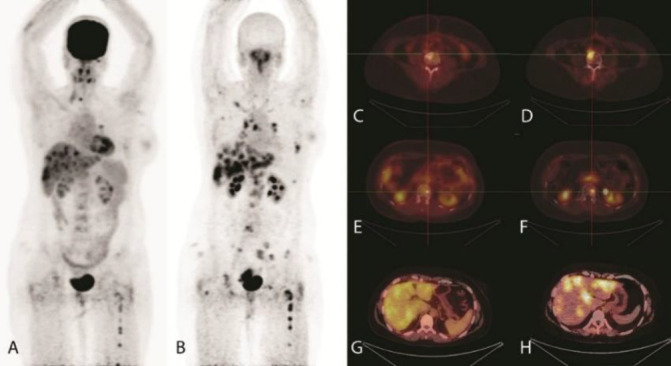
A 35-year-old female, known case of right sided breast cancer. [^18^F]FDG and [^68^Ga]Ga-FAPI-46 PET/CT MIP images (A and B, respectively) demonstrate numerous liver and bone metastases. [^68^Ga]Ga-FAPI-46 images demonstrate more radiotracer avid bone lesions (D, F), which were not present or were less visible in the [^18^F]FDG scan (C, E). Also, Lesion-to-liver ratio was higher in [^68^Ga]Ga-FAPI-46 (H) scan as compared with [^18^F]FDG (G)
